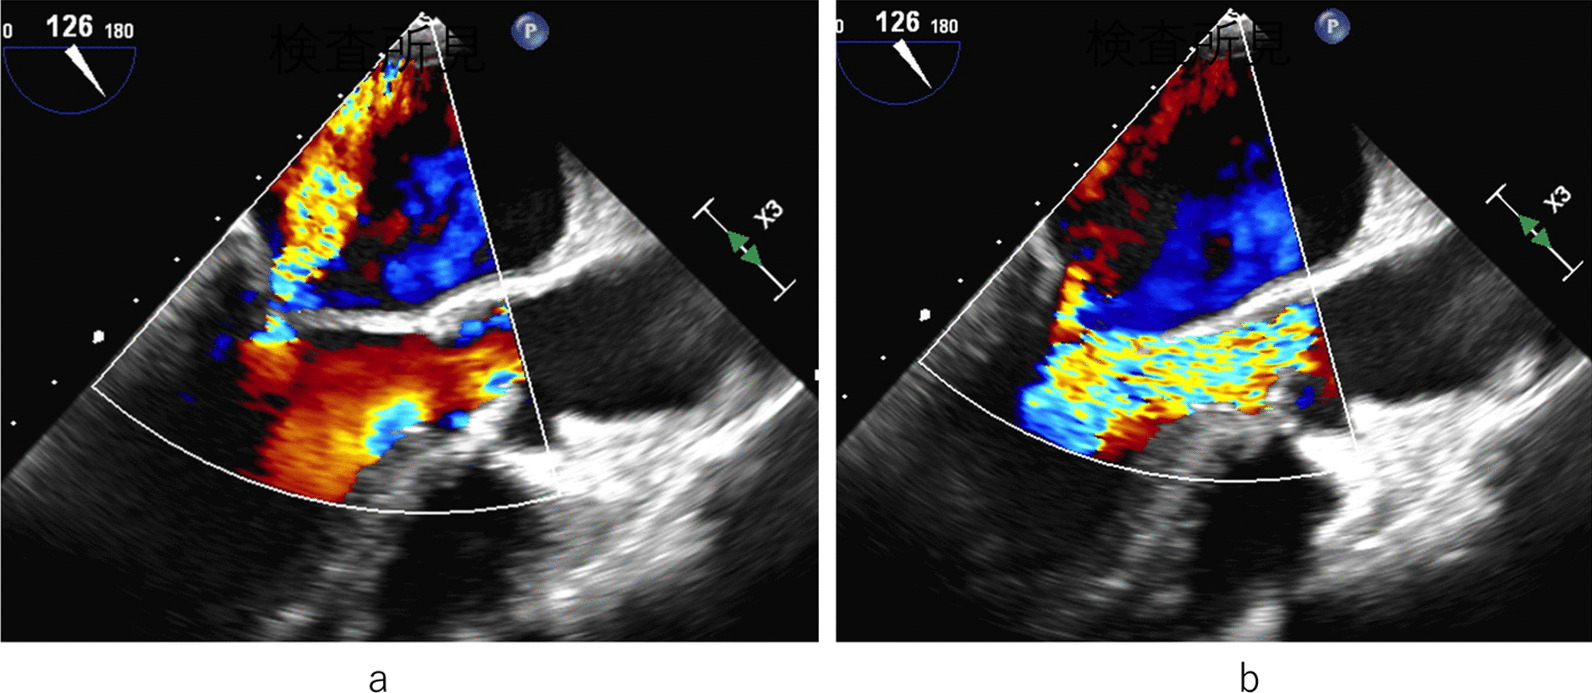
Preoperative transesophageal echocardiography. a Severe mitral valve regurgitation with dilated mitral annulus. b Thickening aortic valve (tricuspid) and severe aortic regurgitation
